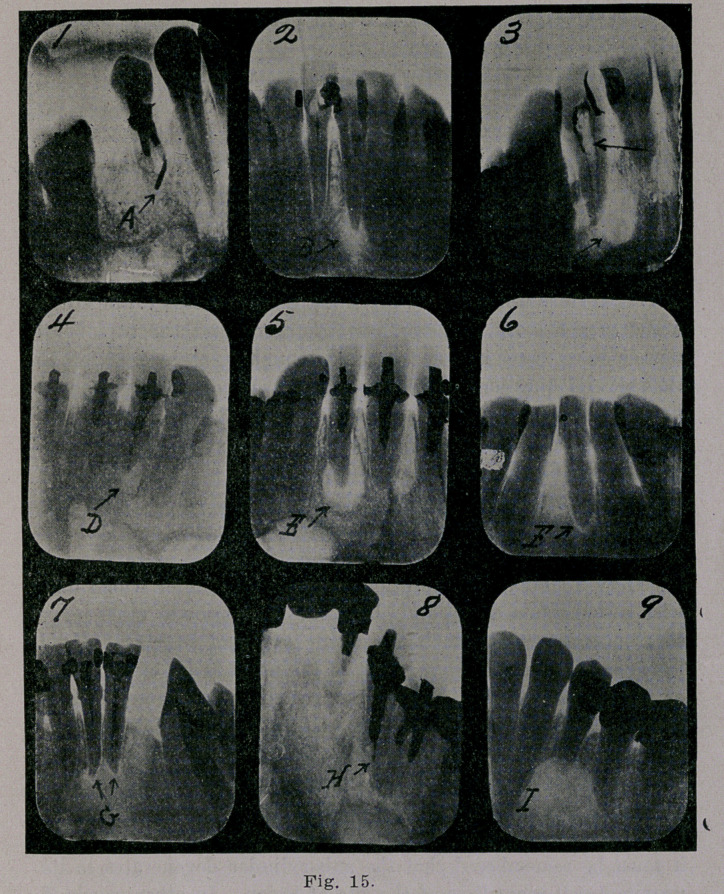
# Indications for the Radiograph in the Study of Oral Conditions
